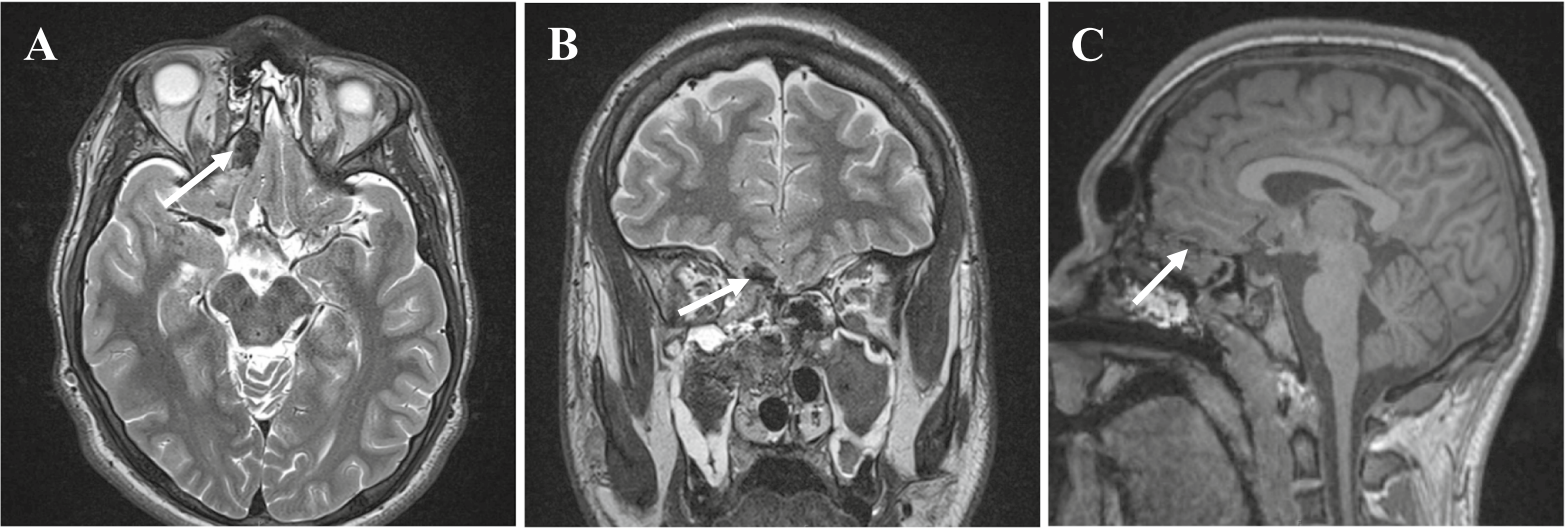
Axial (A) and coronal (B) postoperative T2-weighted and sagittal postoperative T1-weighted non-contrast (C) magnetic resonance images (MRI) obtained the day following surgery showing a gross total resection with otherwise expected post-surgical changes (white arrows)
